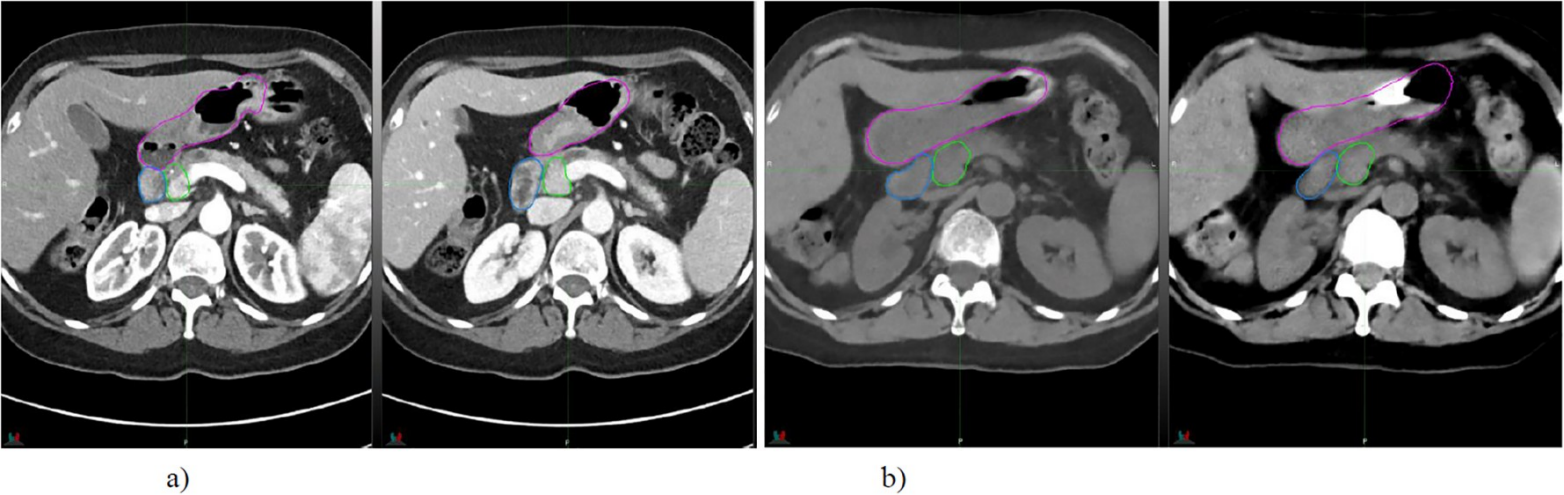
a) two contrast CT images of a patient with one min delay, b) two dual energy CT of the same patient with ~15 seconds delay. Red: stomach, Blue: duodenum and Green: head of pancreas. The shape and direction of the duodenum changed between subsequent minute.
